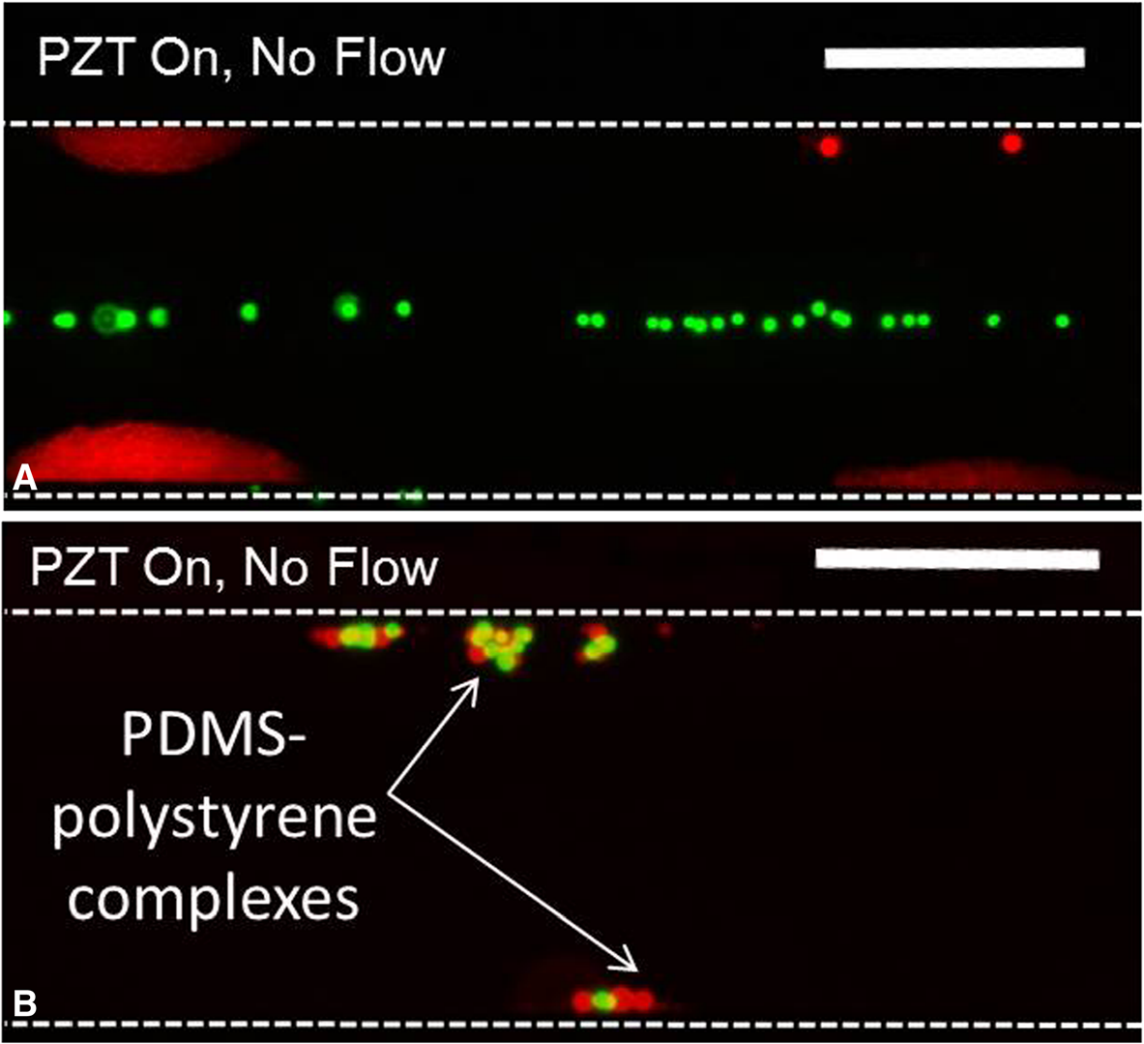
Using NACPs to transport PACPs to the pressure antinode. Fluorescence images demonstrate the ability to use NACPs to transport PACPs to the pressure antinode within an acoustofluidic device. (A) As a negative control, PDMS microparticles (non-biotinylated, encapsulated with Nile Red fluorophore) were mixed with streptavidin polystyrene microparticles (green, 6 μm diameter). The lack of binding between the non-biotinylated PDMS and streptavidin polystyrene particles results in their transport to the antinode and node, respectively. (B) The high affinity between PDMS microparticles (biotinylated, encapsulated with rhodamine B fluorophore) and streptavidin polystyrene microparticles (green, 6 μm diameter) generate particle complexes that transport collectively to the pressure antinode within an ultrasound standing wave. Images acquired in the absence of flow with a 1:10 ratio of polystyrene:PDMS. Dashed lines are included to demarcate the channel boundaries. Scale bars represent 200 μm.
